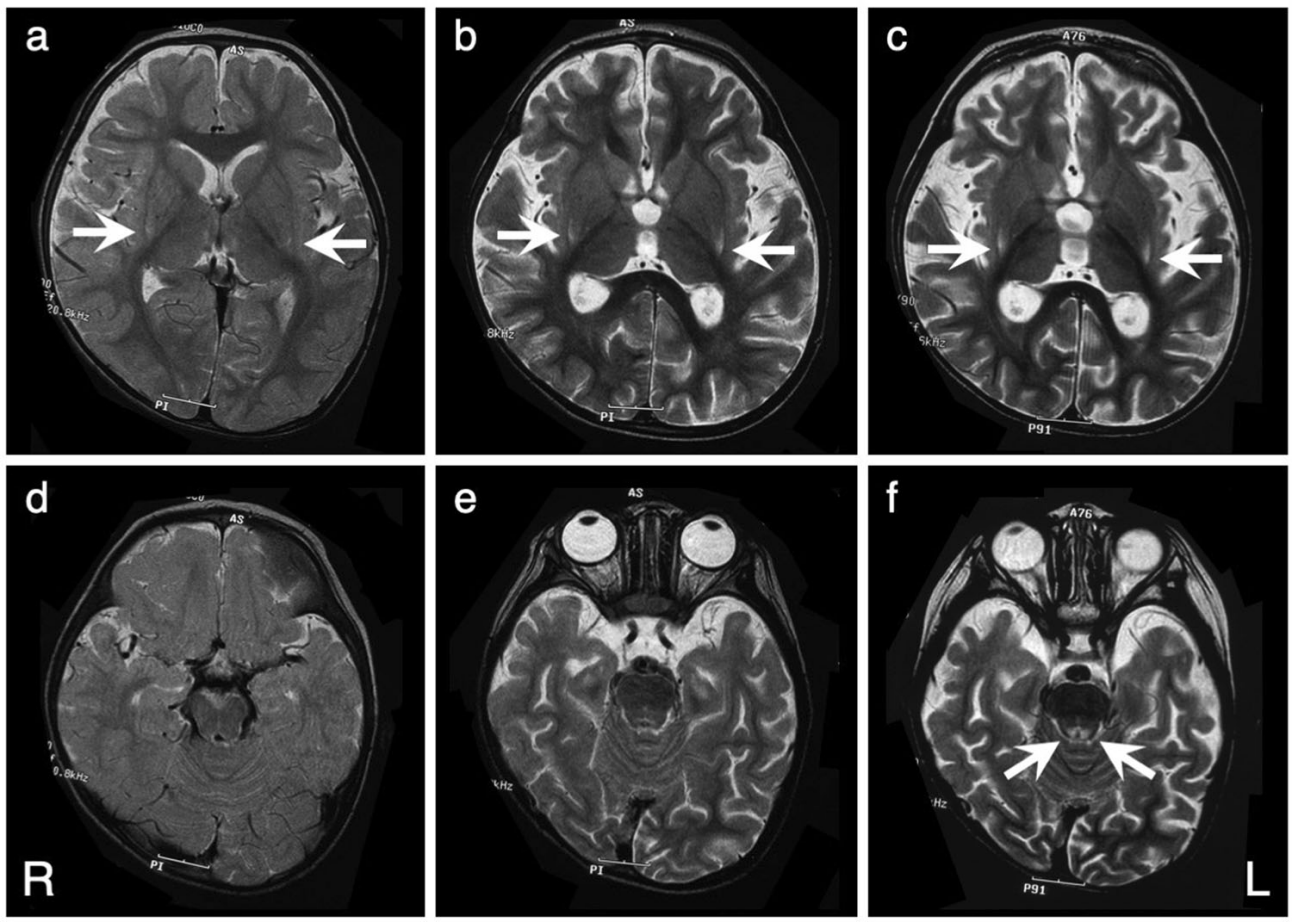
Serial changes in brain T2-weighted MRI findings in a patient with compound heterozygous variants in *NARS2*. Note the bilateral symmetric high signal lesions involving the dorsal putamen (arrows, a–c) and diffuse progressive cerebral atrophy at the age of 1.7 (a, d), 2.3 (b, e), and 3.3 (c, f) years old. Brain stem lesions become apparent at the age of 3.3 years (arrows, f).
